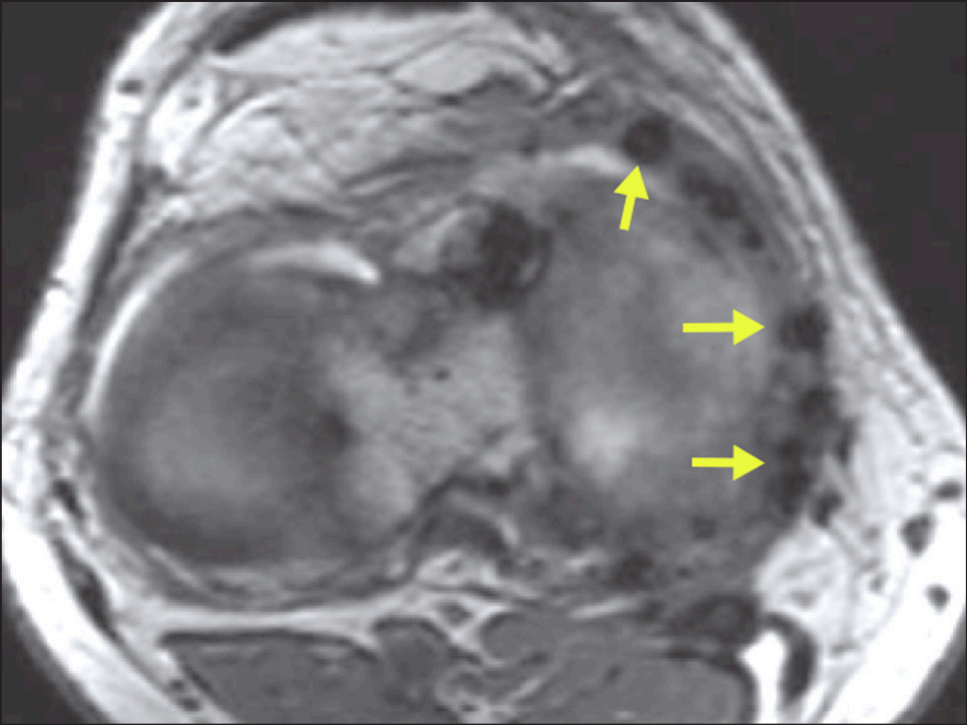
Axial T1-weighted fast spin echo MR-image of a medial meniscal allograft. The whole length of the meniscocapsular junction is obscured by multiple micrometallic artefacts caused by surgical manipulation.
